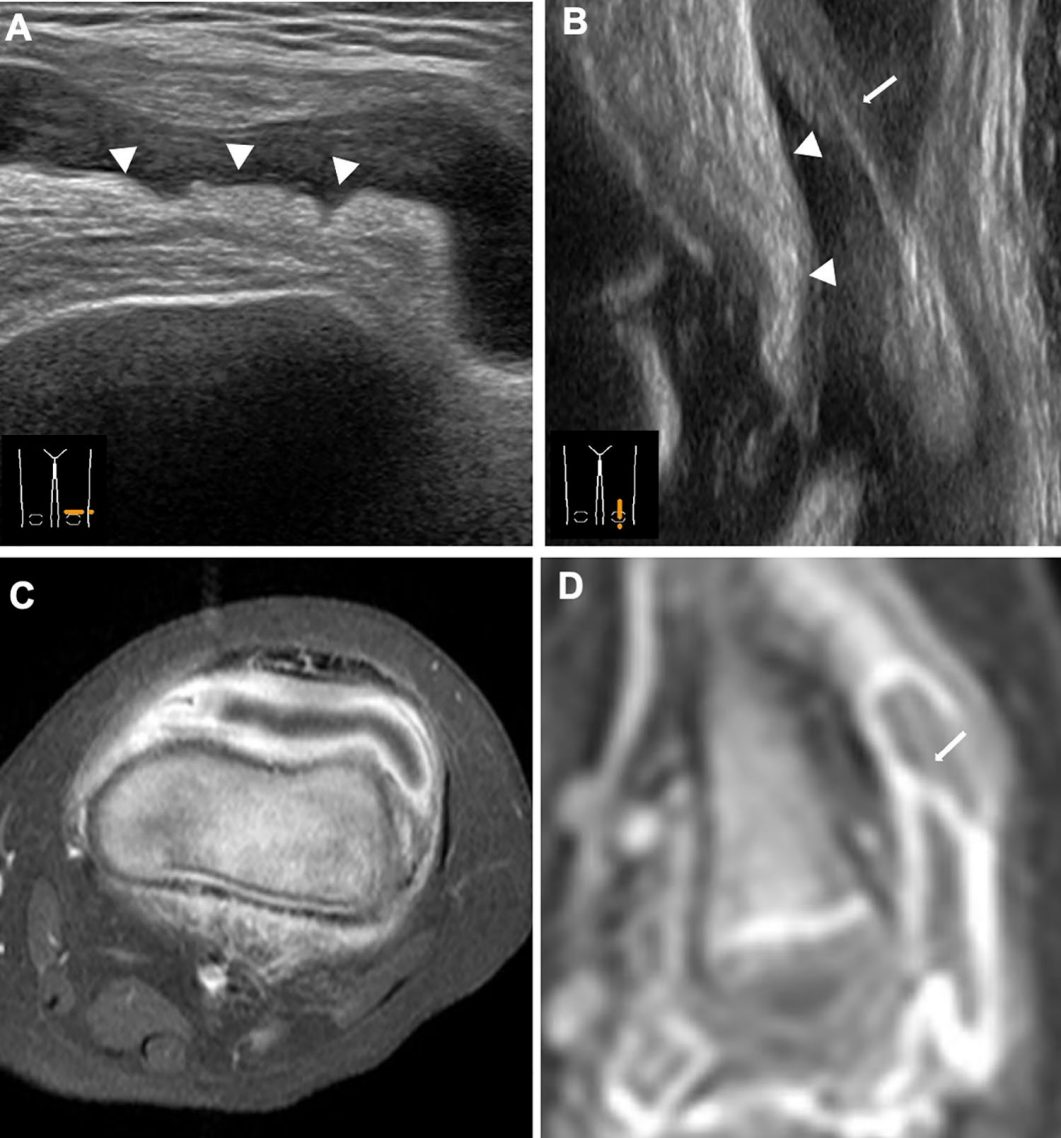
US images for synovitis. A–D. An 18-month-old boy with painful left knee joint. Transverse (A) and longitudinal (B) view of US depicted a heterogeneously thickened, partially wavy synovium (arrowheads, A, B) and increased joint fluid, corresponding to those of post-contrast enhanced axial MRI (C) and the late phase of dynamic contrast-enhanced sagittal MRI (D), respectively. Note thickening of the suprapatellar plica due to its synovial thickening (arrows, B, D)
